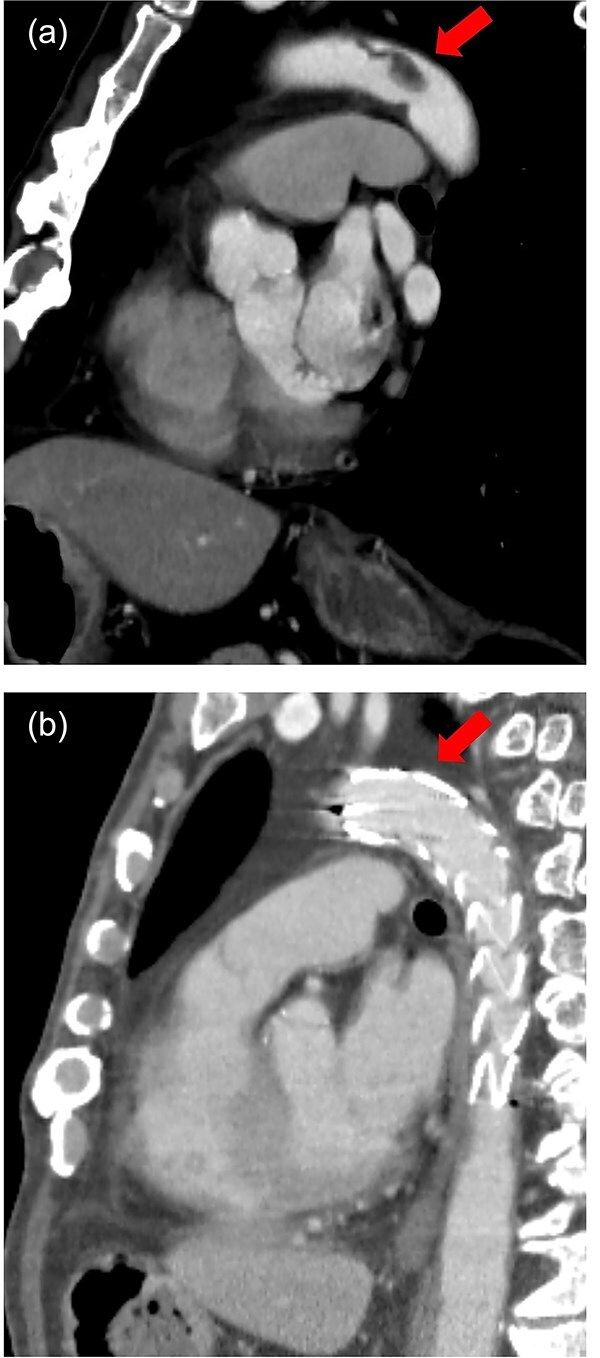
Sagittal view of contrast-enhanced CT. (a) Preoperative. A pedunculated intra-aortic thrombus is attached to the distal arch. (b) Postoperative. Thoracic stent graft is implanted in the distal arch with successful exclusion of the aortic thrombus.
